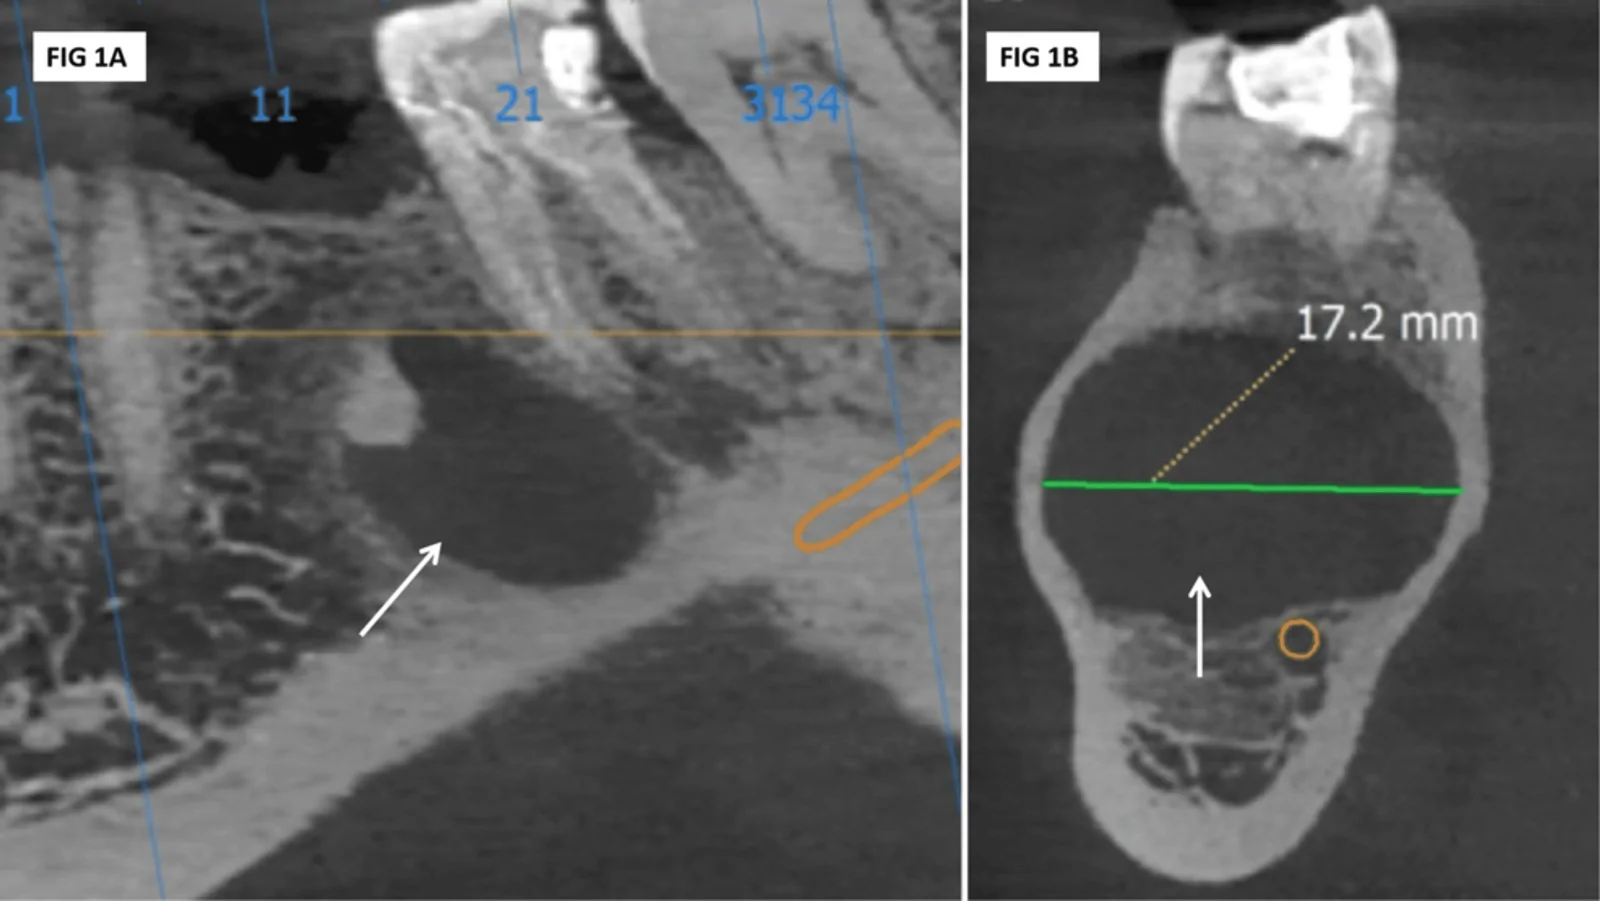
Pre-operative radiographic analysis by cone beam computed tomography (CBCT) (A) The arrow shows radiolucency of the cystic lesion in panoramic view. (B) The arrow shows radiolucency of the cystic lesion in the sagittal view.
